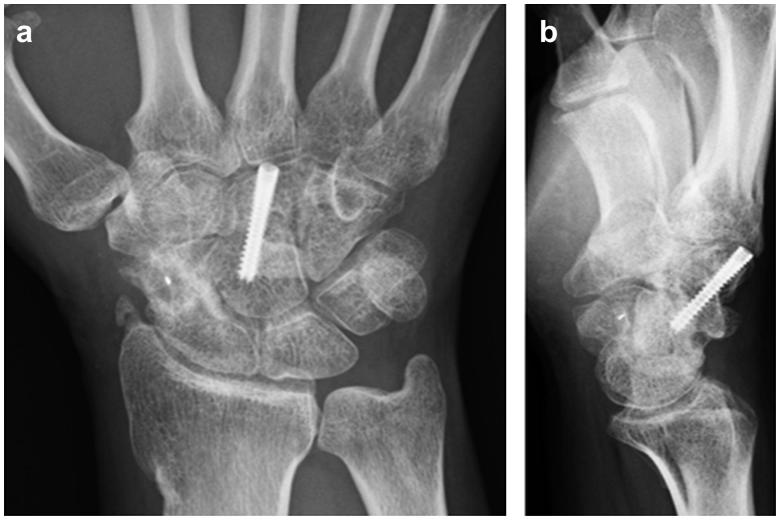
Postoperative radiographic assessment of the hand. Radiographs showing the healing of both nonunions without evidence of arthritic changes.
